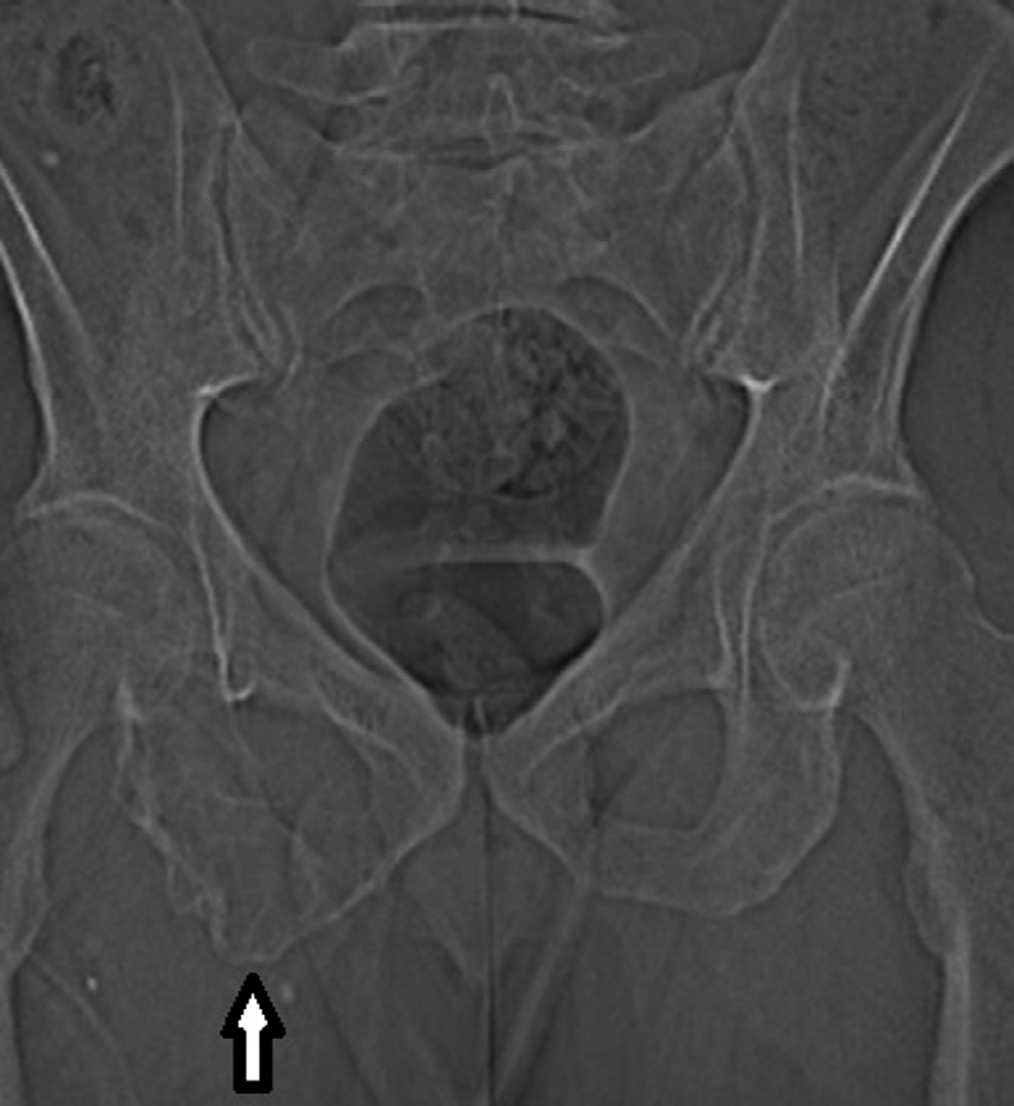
Pelvic XRays was performed (Figure 1) showing an osteolytic lesion of the right ischio pubic ramus.
